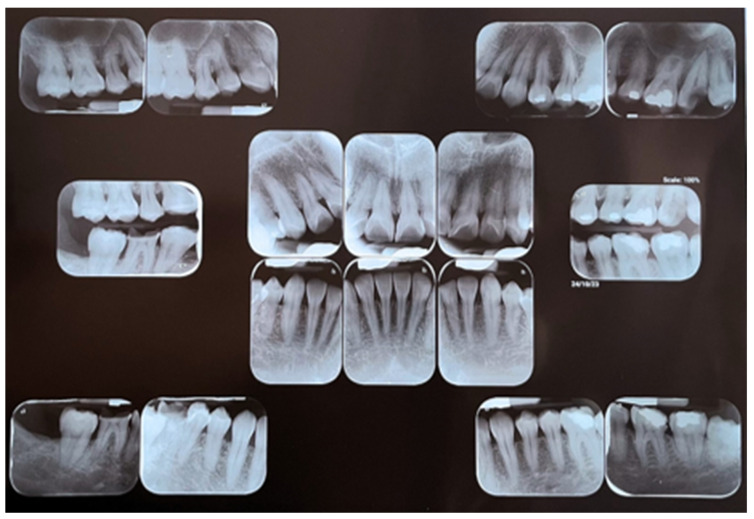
Periapical series where mild loss of bone crest height in lower right molars is observed.
